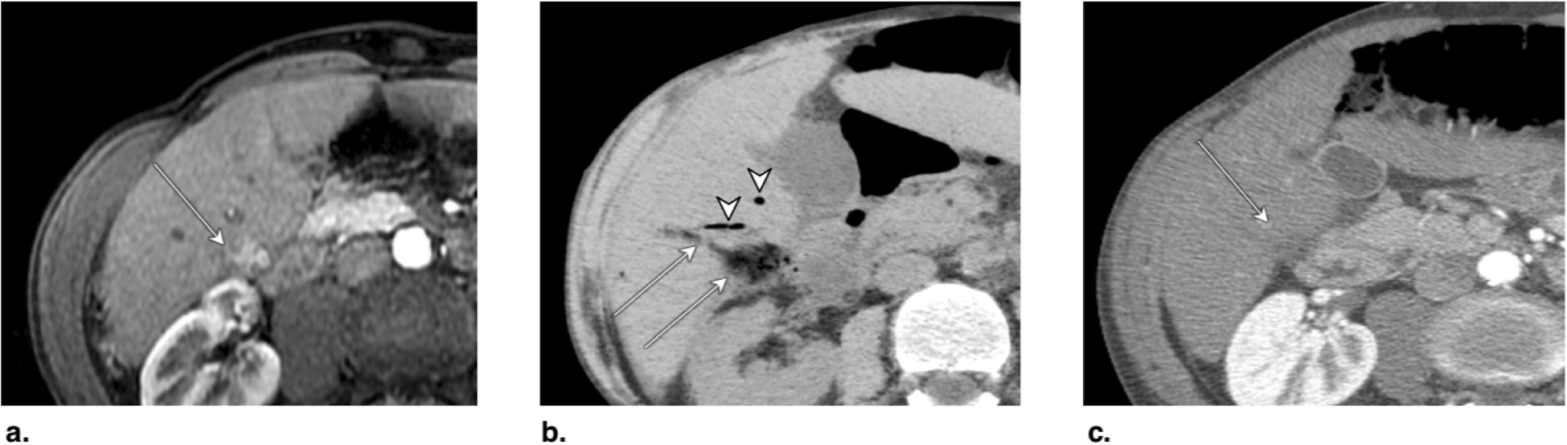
a Axial T1-weighted MR in late arterial phase (AP) obtained pretreatment: image shows nonrim arterial phase hyperenhancement of a 2-cm LR-4 observation (arrow). b Axial-unenhanced CT MinIP obtained immediately post percutaneous ethanol ablation (PEA): image shows pneumobilia (arrowheads), ablation cavity, and needle trajectory (arrows). c Axial CT in late AP obtained 4 months post PEA: image shows no enhancement and cavity retraction (arrow). The treated observation is categorized LR-TR Nonviable
